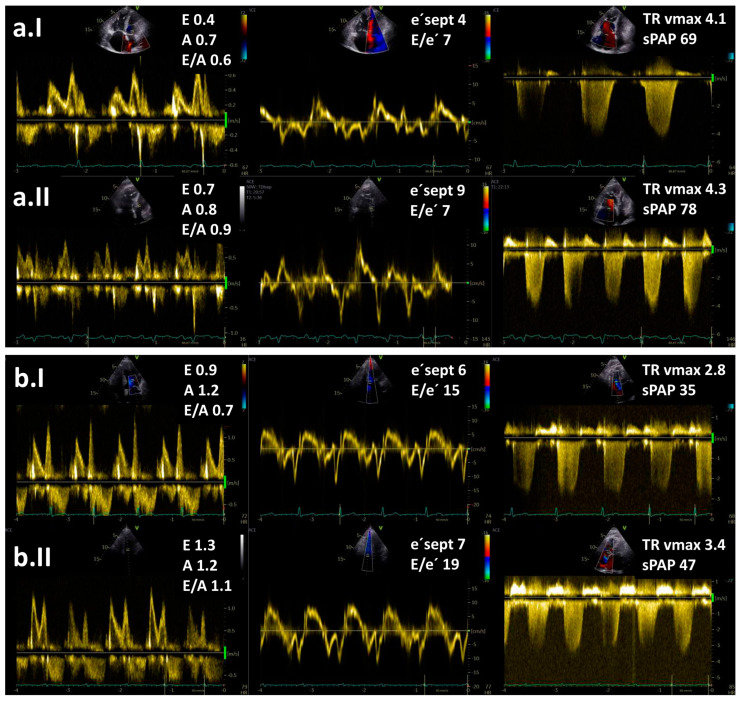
Resting (I) and exercise stress echocardiography (II) in two patients with pulmonary hypertension (PH): (a) a patient with pre-capillary PH and (b) a patient with post-capillary PH. Maximal tricuspid regurgitation velocity (TR vmax) is elevated in both patients. Note an increase in E/e′ ratio at low-level exercise from 15 to 19 in a patient with post-capillary PH but no increase in a patient with pre-capillary PH. Legend: sPAP—systolic pulmonary arterial pressure.
